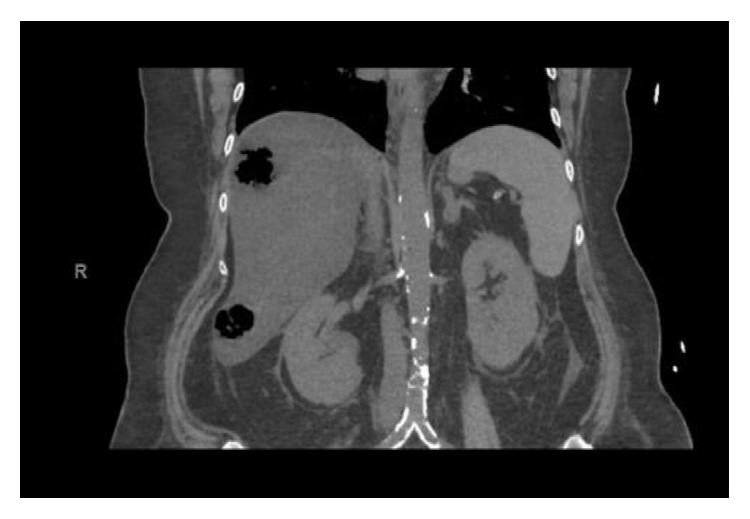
CT abdomen/pelvis demonstrating multiple abscesses in the liver with air fluid levels.
